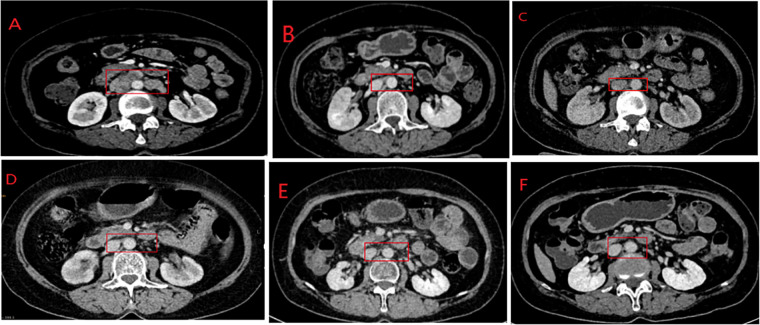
Abdominal CT scans of the patient. (A) CT showed multiple enlarged lymph nodes in the retroperitoneum, the largest one of which measures approximately 22 mm; it was regarded as the target lesion. (B) After two cycles of the combination of EP and camrelizumab, CT demonstrated shrunken the multiple lymph nodes in the retroperitoneum, the larger one has a short-axis of approximately 7 mm. (C) 5 cycles of camrelizumab + EP, CT showed that retroperitoneal lymph nodes further size reduction, the maximum diameter was approximately 6 mm. (D) Monotherapy with camrelizumab for sustained immunotherapy, CT scan revealed that the largest short axis diameter of the retroperitoneal lymph node to be 5 mm. (E) After 15 cycles of camrelizumab, the retroperitoneal lymph nodes remain at 5 mm in size. (F) Abdominal CT showed the target lesion was maintained at 5 mm in size. Red box: Retroperitoneal lymph nodes.
